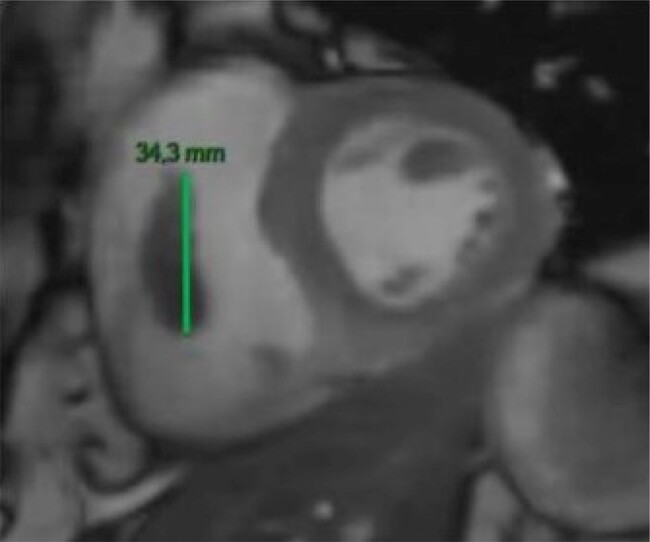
Cardiac magnetic resonance image showing right intraventricular thrombus.
